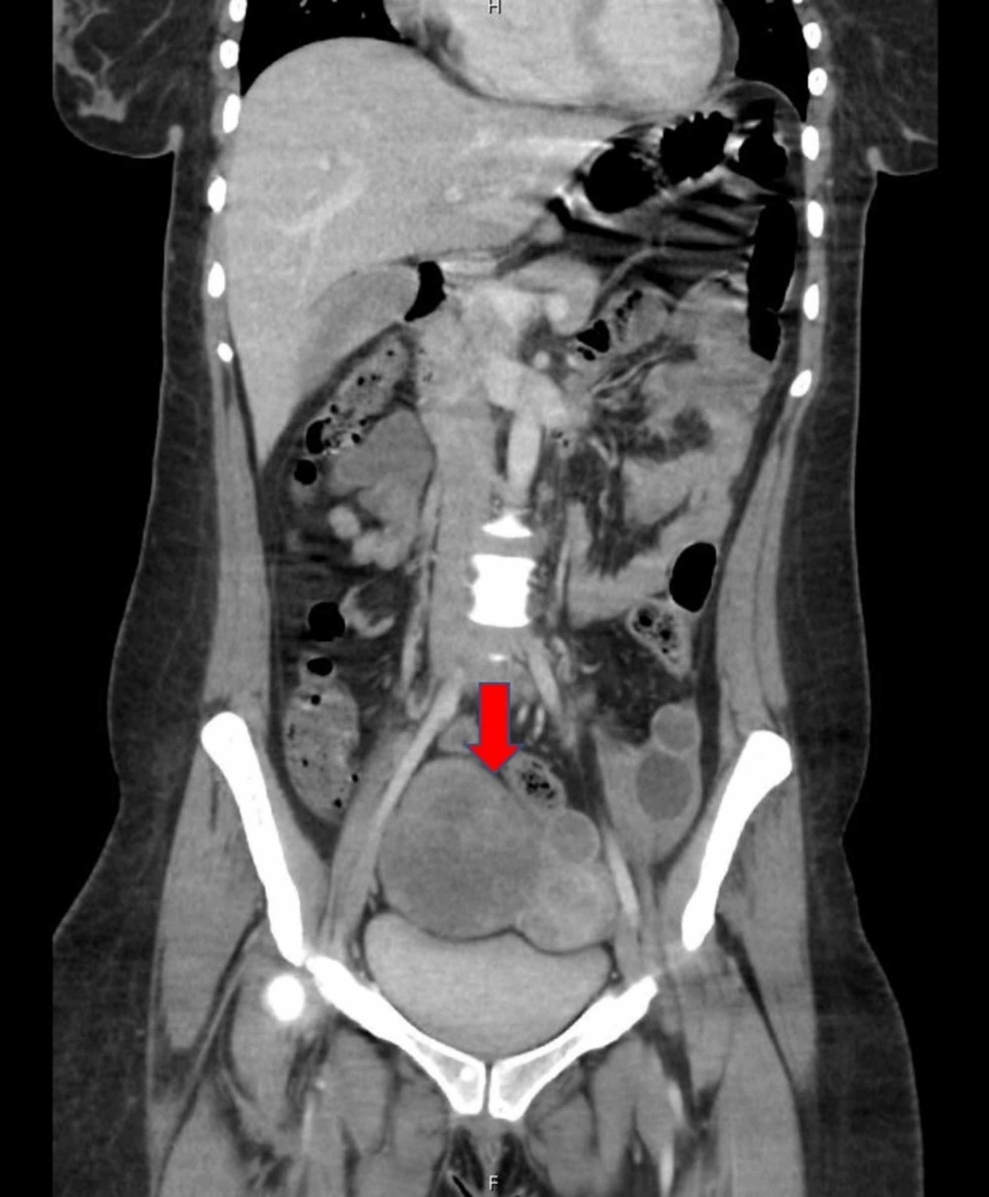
Coronal view of the abdomen and pelvis CT demonstrating a 9.5 cm solid and cystic right adnexal mass.
